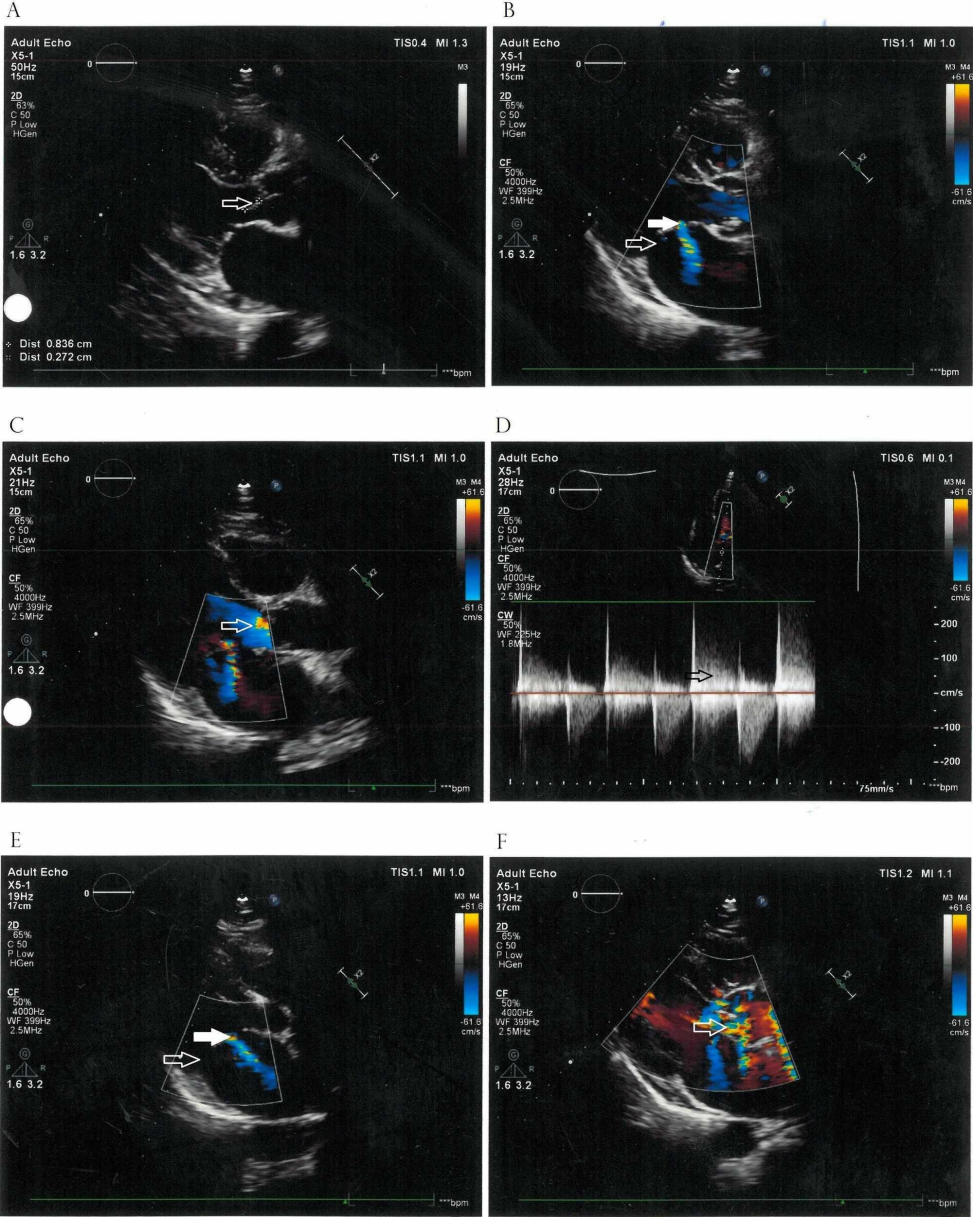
Transesophageal echocardiography showing vegetations on the aortic valve. (A) Hollow arrow shows vegetations on the ventricular side of the aortic valve. (B) Solid arrow shows regurgitation through a perforated anterior mitral leaflet. Hollow arrow shows the normal position of the blood flow through the mitral valve. (C) Hollow arrow shows aortic regurgitation. (D) Hollow arrow shows aortic regurgitation. (E) Solid arrow shows regurgitation through a perforated anterior mitral leaflet. Hollow arrow shows the normal position of the blood flow through the mitral valve. (F) Hollow arrow shows aortic regurgitation.
